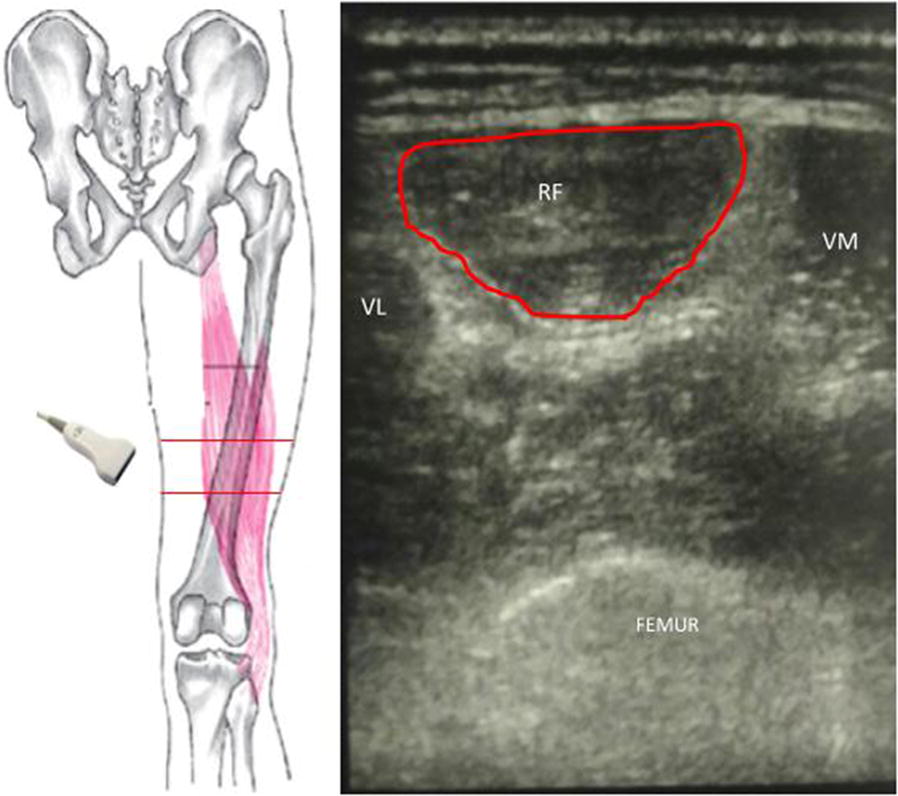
Muscle cross-sectional area. This figure depicts the cross-sectional area of the rectus femoris perpendicular to its longitudinal axis. The quadriceps femoris is a group of muscles composed by three vastus muscles (medialis, intermedius, and lateralis) and the rectus femoris which presents a proximal insertion in the anterior inferior iliac spine and other insertion in the supra-acetabular sulcus. Left side: standardized level of ultrasound scan of the lower limb; in the supine position, the probe should be placed at 2/5 of an imaginary line between the anterior parts of the thigh from the anterior inferior iliac spine to the midpoint of the proximal border of the patella. Right side: the figure depicts the cross-sectional area (red circle) of the rectus femoris (RF) perpendicular to its longitudinal axis. *VI* vastus intermedius, *VM* vastus medialis, *VL* vastus lateralis
